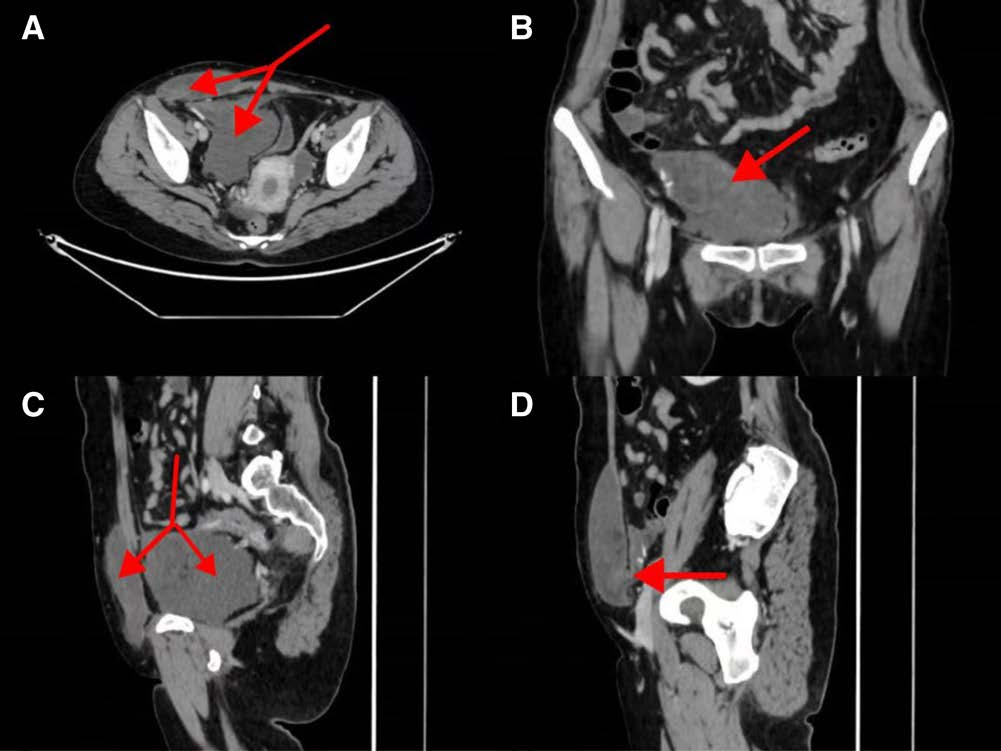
Abdominal computed tomography (CT) showed an irregular mass (arrows) from the right lower abdominal wall to the right side of the pelvis, with uneven density, fat density shadows, and strip vascular shadows. The volume was approximately 15 cm × 12 cm.
